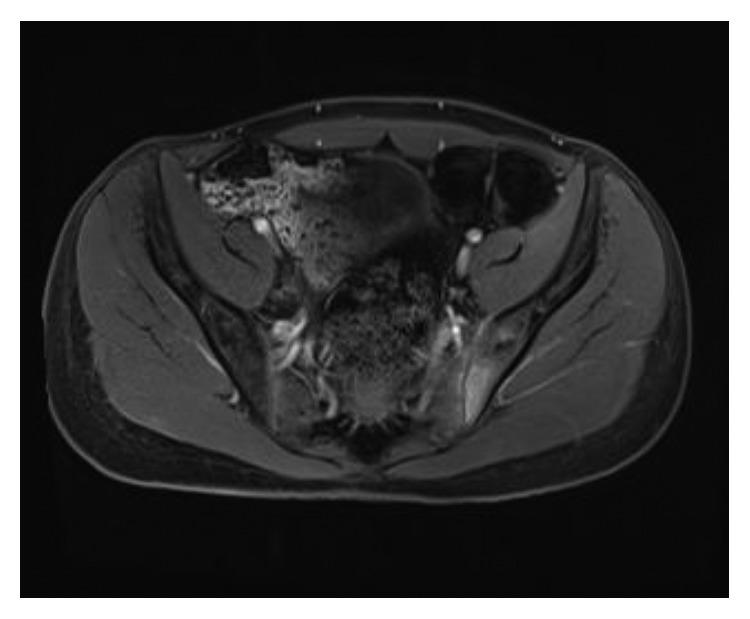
MRI of the hip showing axial T1-weighted postcontrast enhancement involving the visualized parts of the left ilium and sacrum at the sacroiliac joint.
